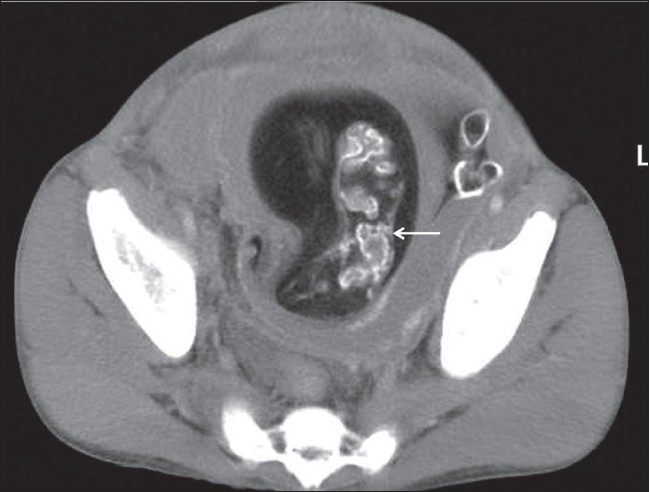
Axial contrast-enhanced CT scan (inferior to B) shows the rudimentary spine (arrow) of the smaller fetus
